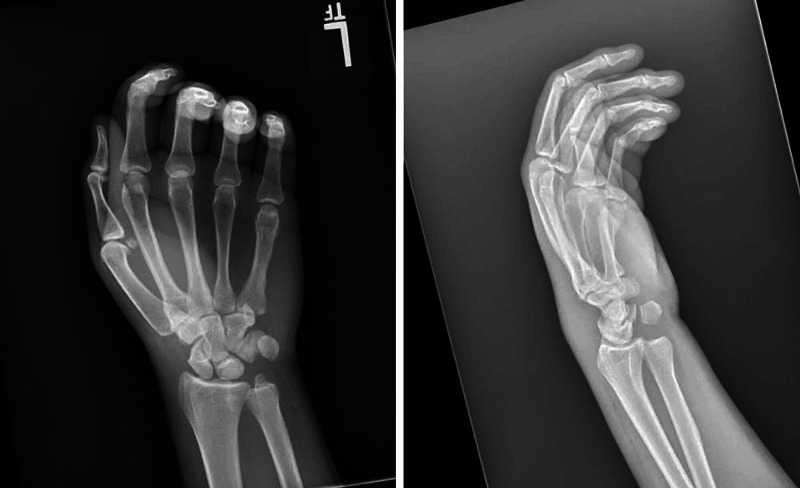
Initial posteroanterior (left) and lateral (right) radiographs of the injury
